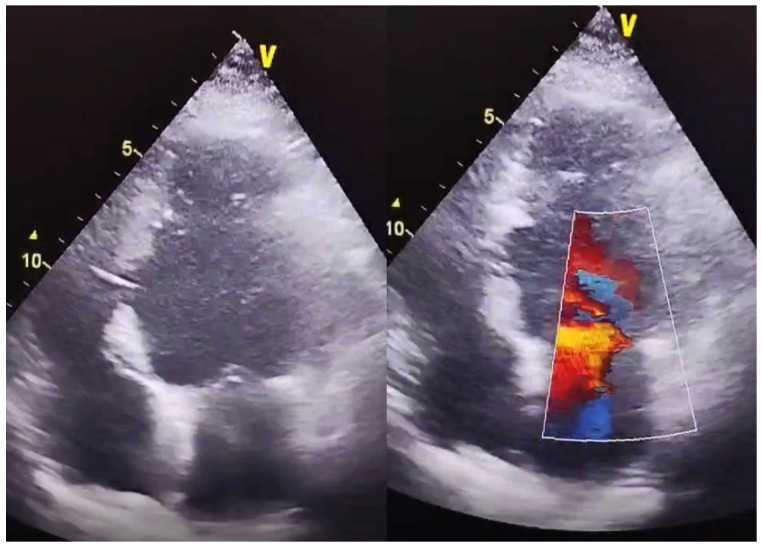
Postoperative follow-up echocardiography with no residual shunt.
